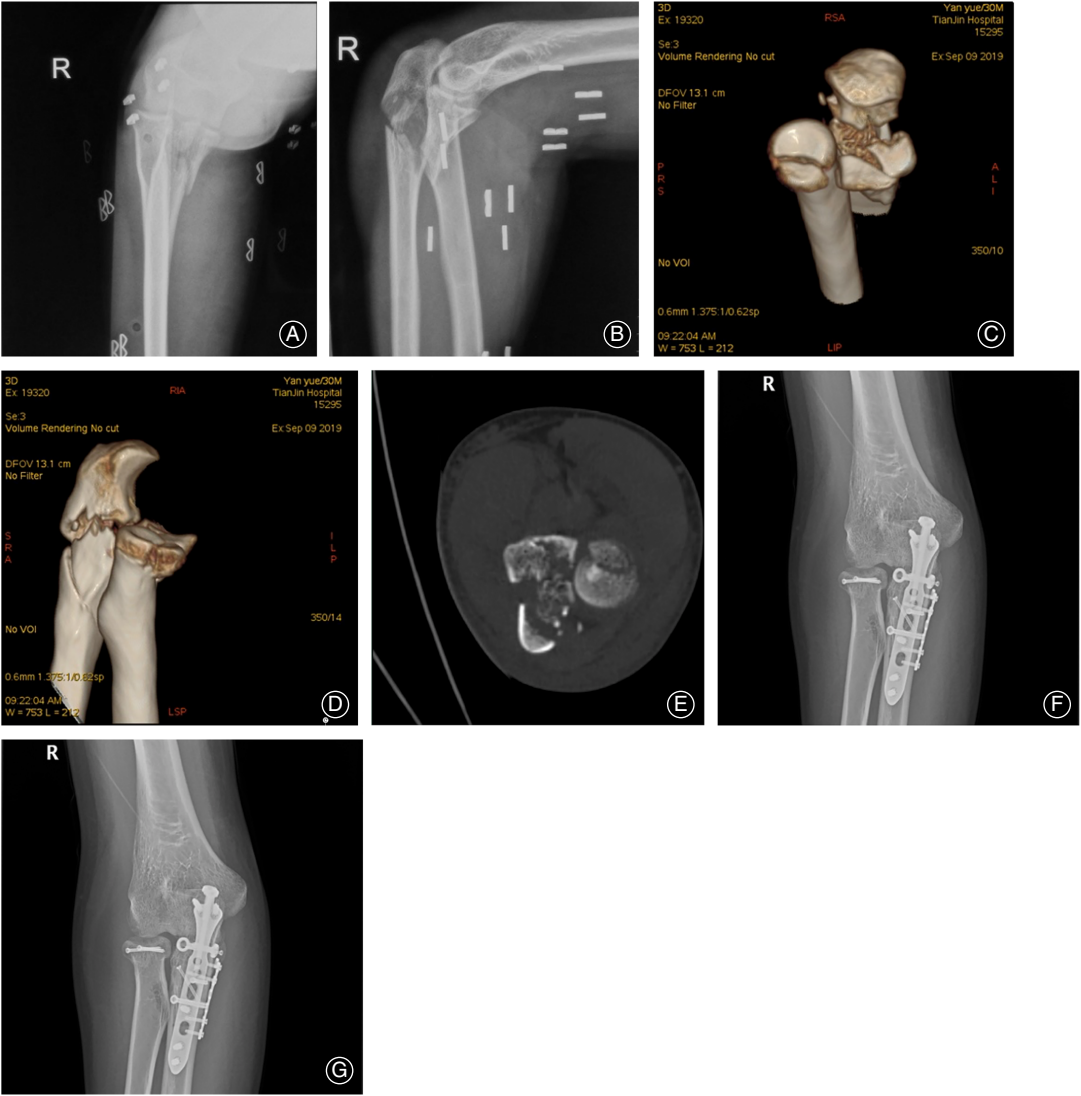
Patient No. 27. A 30‐year‐old man fell from the standing height and injured his right dominant elbow. (A, B) Initial anteroposterior and the lateral radiograph showed proximal ulna fracture and radial head fracture. Standard anteroposterior and the lateral radiographs cannot be obtained due to severe pain in the patient. (C, D, E) A computed tomography image showed that an Adams Type IV(AL)b fracture of the coronoid process. (F, G) The anteroposterior and lateral radiographs that were obtained following the operative treatment.
